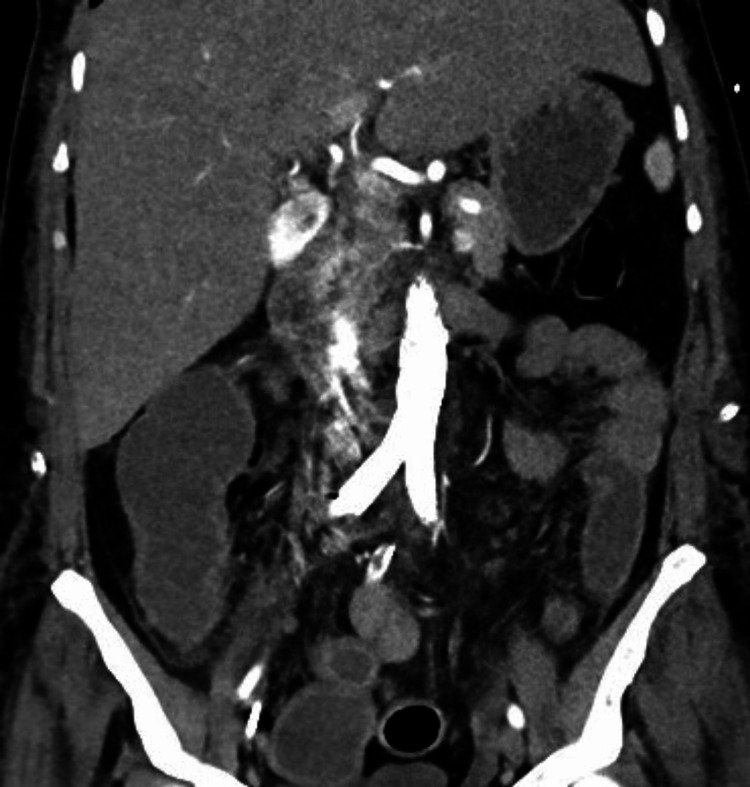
Control computed tomography angiography, coronal image, after the procedure, coronal view, showing a good procedure result with no endoleak and extravasation
